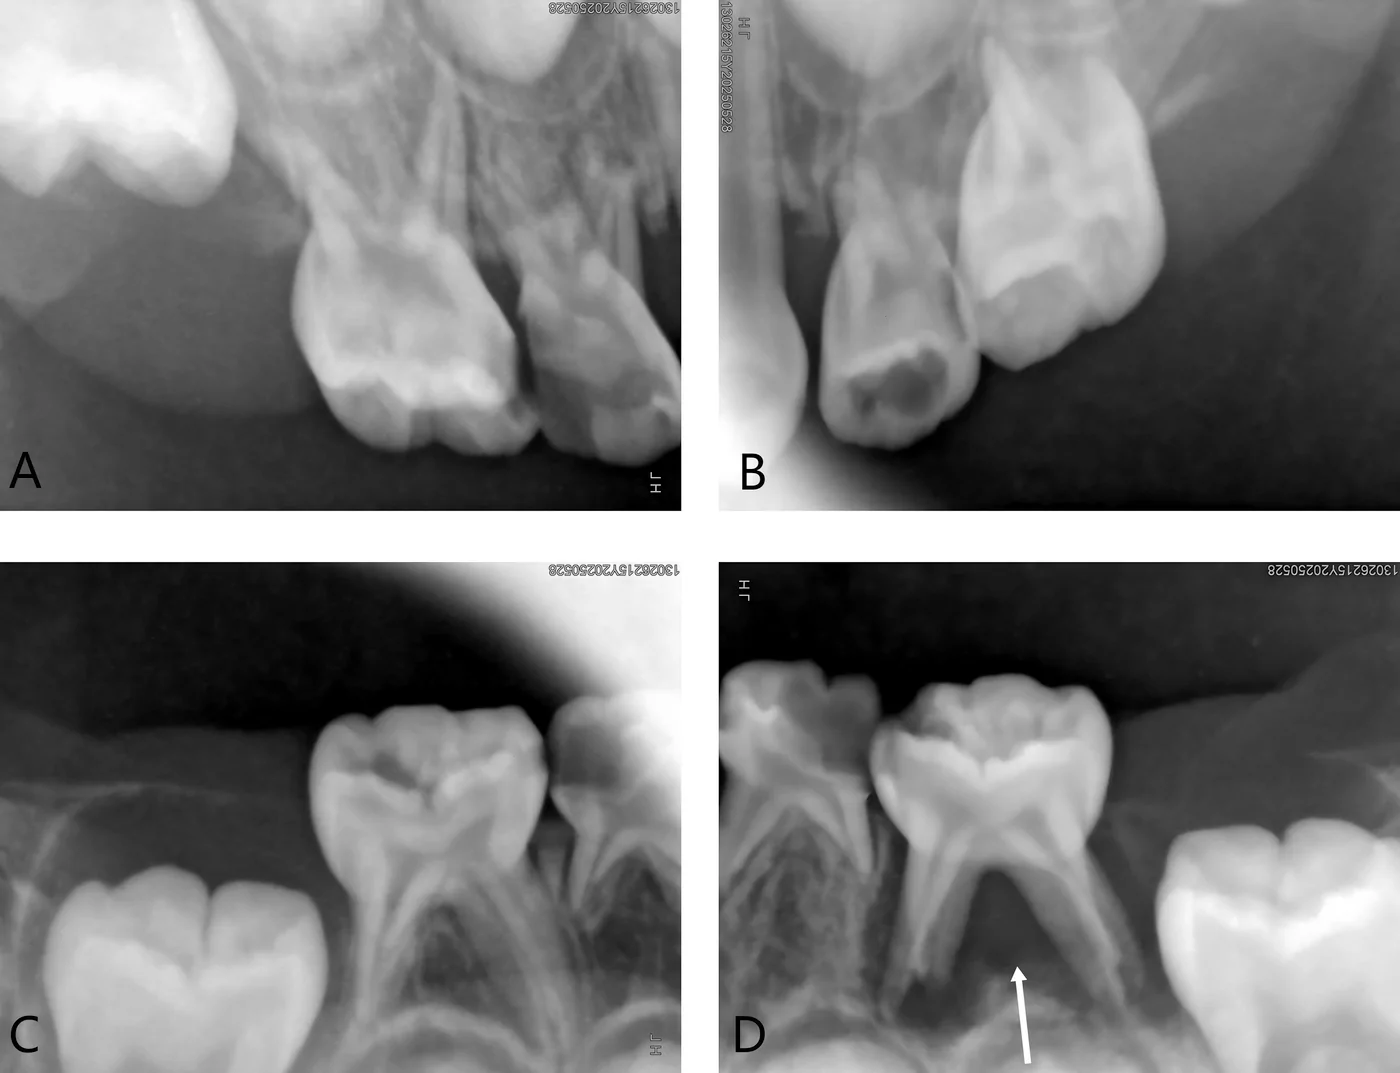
Periapical radiographs obtained after induction of general anesthesia. (A-C) Multiple cavitated carious lesions involving the primary molars. (D) Tooth 75 showed root resorption and periapical radiolucency. The arrow indicates the radiolucent lesion, with an incomplete corticated border of the developing permanent teeth.
